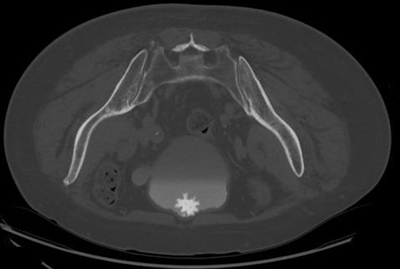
Axial delayed contrast phase CT scan in prone position. A ‘Jackstone’ is seen anteriorly in the bladder. Also notice a diverticulum at the left lateral bladder wall.
